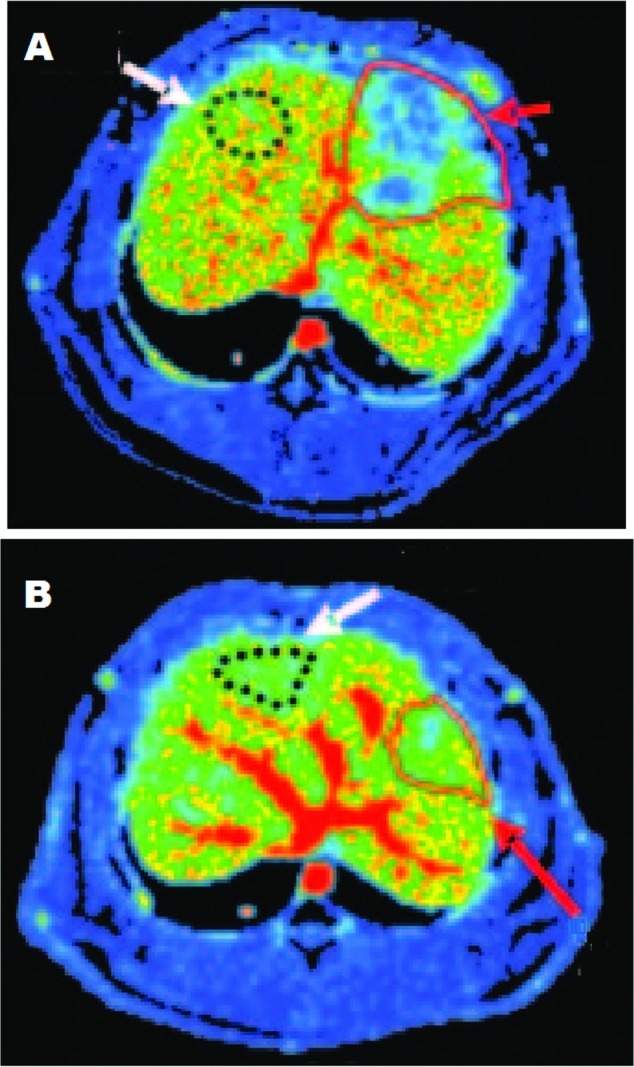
HBF maps obtained by CT perfusion (A) 1 week before and (B) 6 weeks after therapy (the normal tissue: white arrows; tumors: red arrows). HBF, hepatic blood flow; CT, computed tomography.
